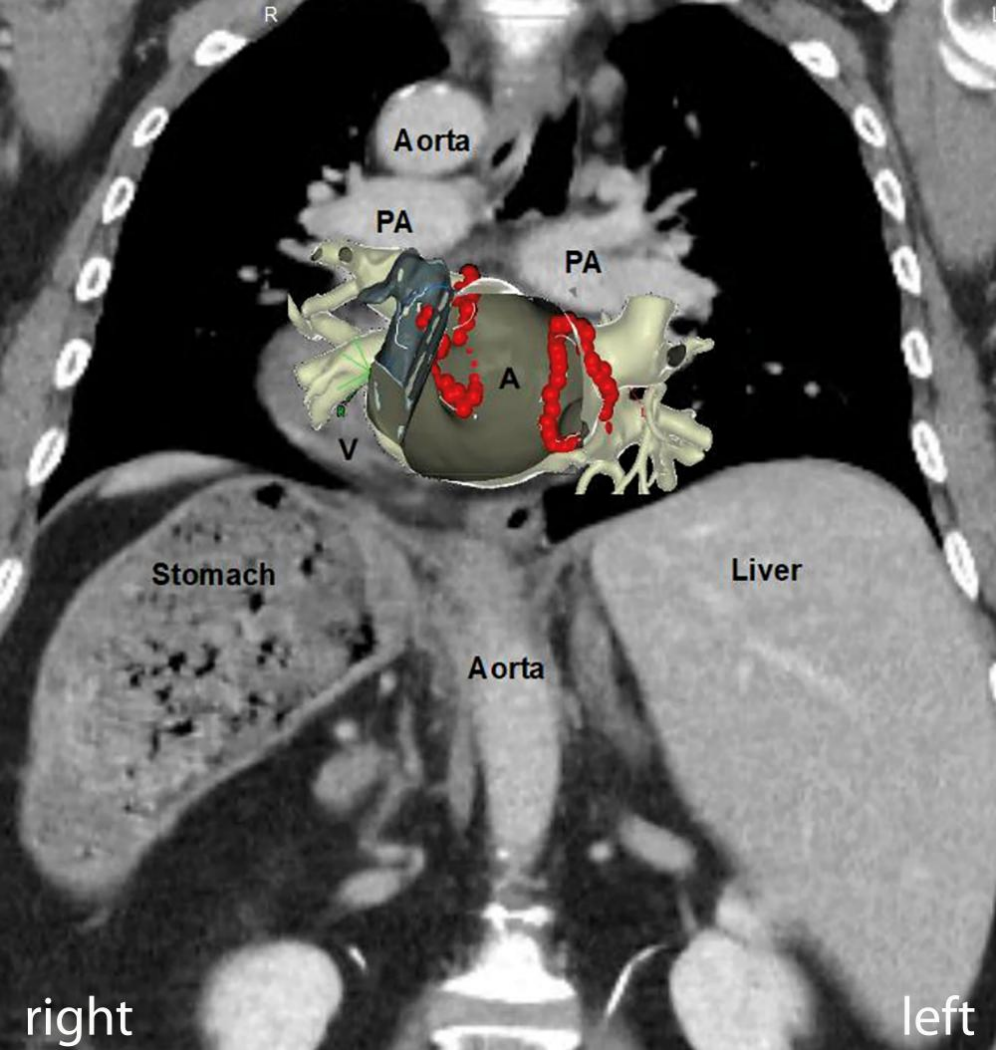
CT with overlaying Carto geometry. A, atrium; V, ventricle; PA, pulmonary artery.
